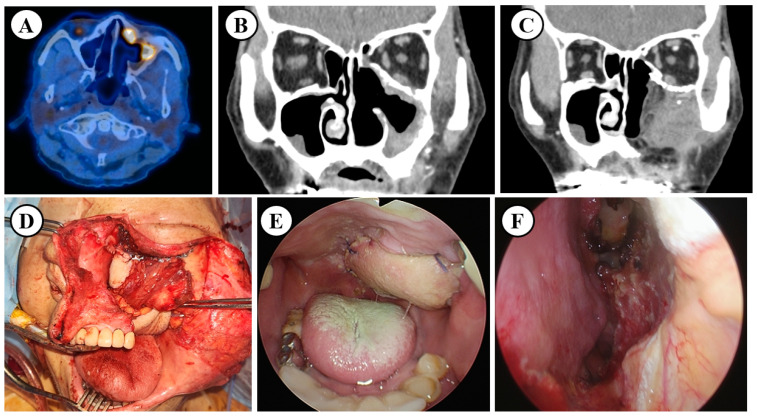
Clinical images of 79 years old male who had 3D printing-guided salvage maxillectomy after the failure of definitive chemoradiation. (A) PET-CT revealed maxillary sinus cancer in anterolateral wall of sinus. (B) Preoperative CT coronal image showed maxillary sinus cancer involving orbital inferior wall, inferolateral wall of maxillary sinus. (C) Postoperative CT image revealed well-reconstructed orbital plated and anterolateral thigh free flap at 3 months after the surgery. (D) Intraoperative image of maxillectomy defect and reconstruction with anterolateral thigh free flap and prefabricated orbital mesh plate. (E,F) Endoscopic image of hard palate and nasal cavity reconstructed by anterolateral free flap at 3 months post operation.
